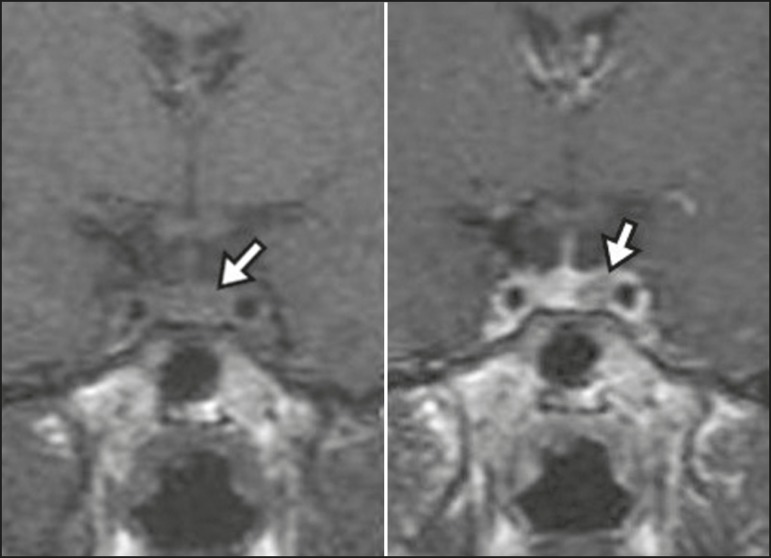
Dynamic contrast-enhanced coronal T1-weighted MRI sequences showing a
